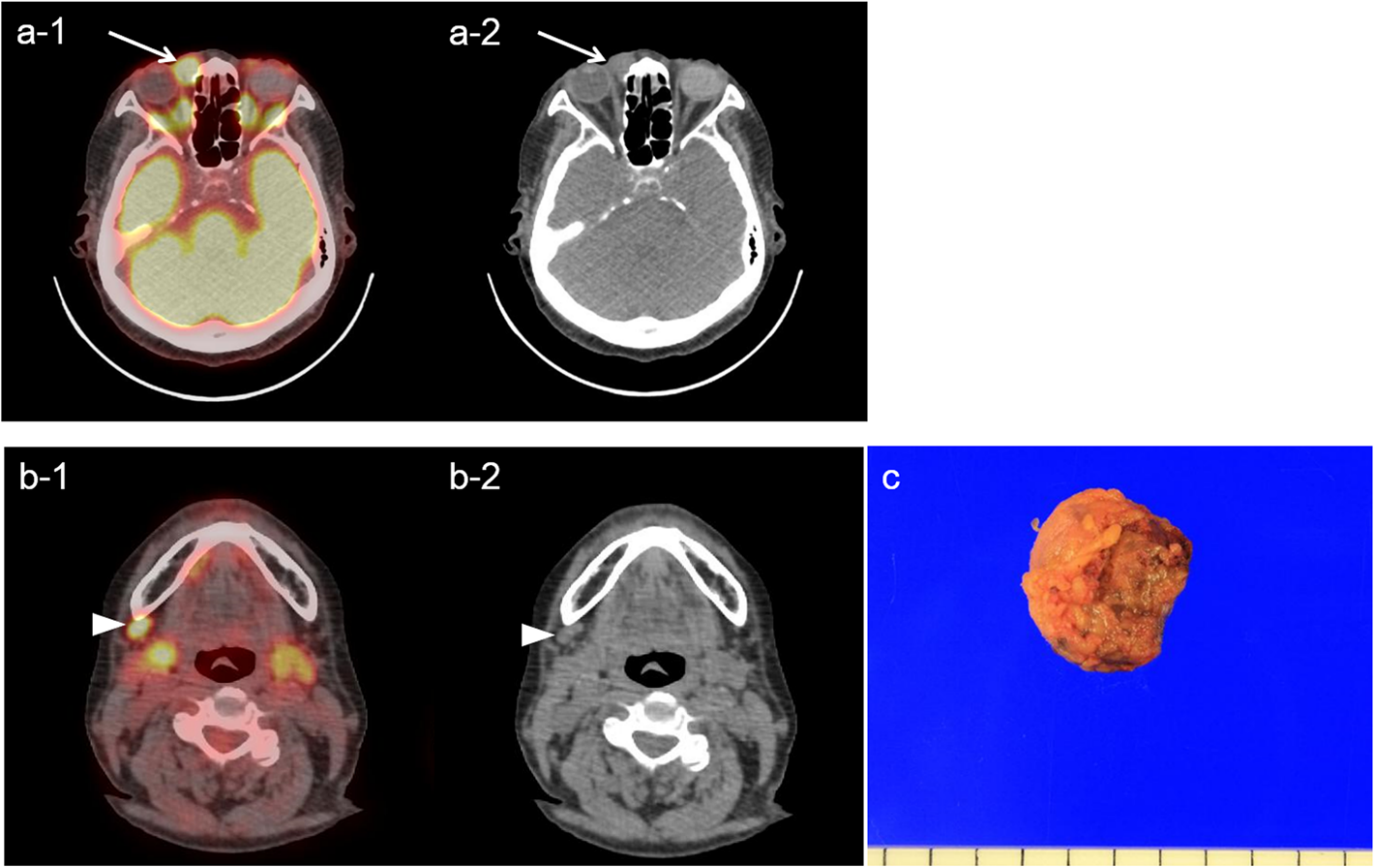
OAML in right orbit (lacrimal sac) with cervical LN involvement. A 72-year-old female had MALT lymphoma in right orbit. a Focal FDG uptake was seen in right lacrimal sac (arrows, SUVmax 10.5) on FDG PET/CT scan. b Another focal FDG uptake was noted in right cervical level I (arrowheads, SUVmax 10.8). c Surgical excision of right cervical level I LN was done, and the final pathology was MALT lymphoma
